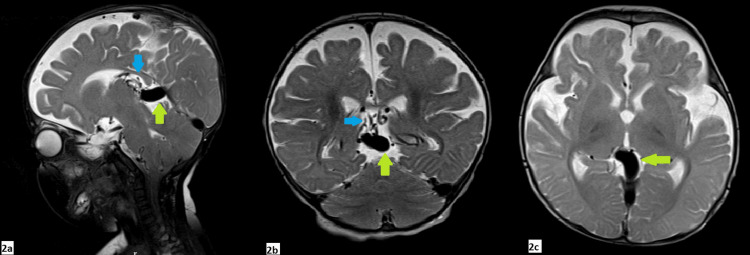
Sagittal (2a), coronal (2b), and axial (2c) 1.5T MR images of the abdomen showed a characteristic appearance of VGAM with a large flow void in the midline, posterior to third ventricle representing enlarged median prosencephalic vein of Markowski of maximum diameter 7 mm (green arrows). Arterial feeders were seen along the anterior wall of the vein (blue arrows).
